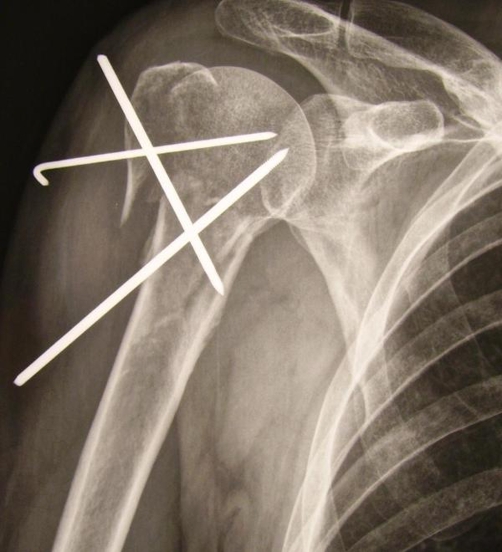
Post-operative radiograph of the three-part fracture of the right humerus head be fixed with three percutaneous Steinman wires.
